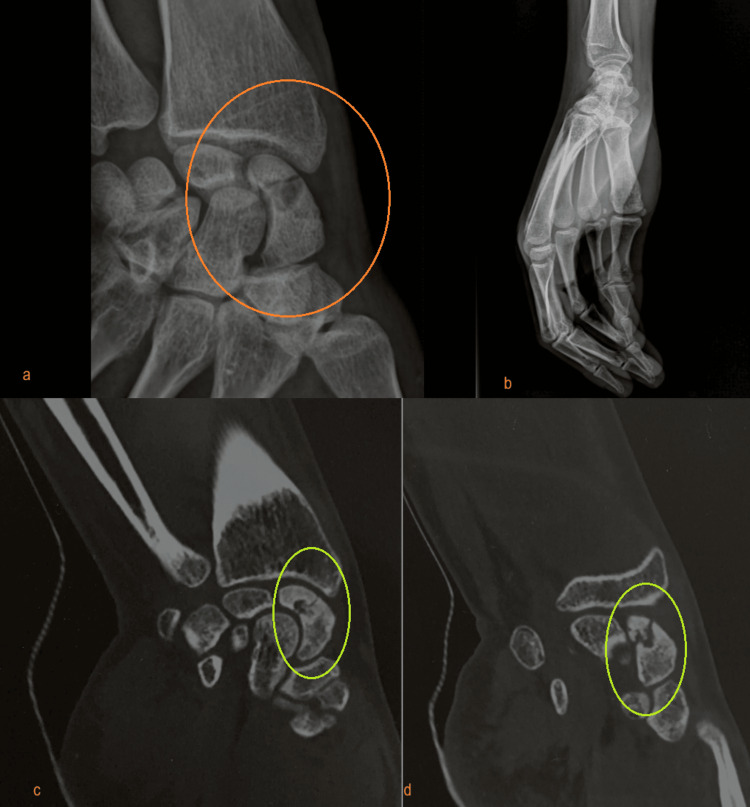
Radiographs and CT scan showing a stage IIA scaphoid nonunion with proximal pole sclerosis suggestive of avascular compromise (a) Posteroanterior and (b) lateral wrist radiographs demonstrating a stage IIA scaphoid nonunion according to the Alnot and Bahri classifications (orange circle). CT scans (c, d) showing a persistent fracture line with sclerotic margins and increased density of the proximal fragment, suggestive of avascular compromise.
